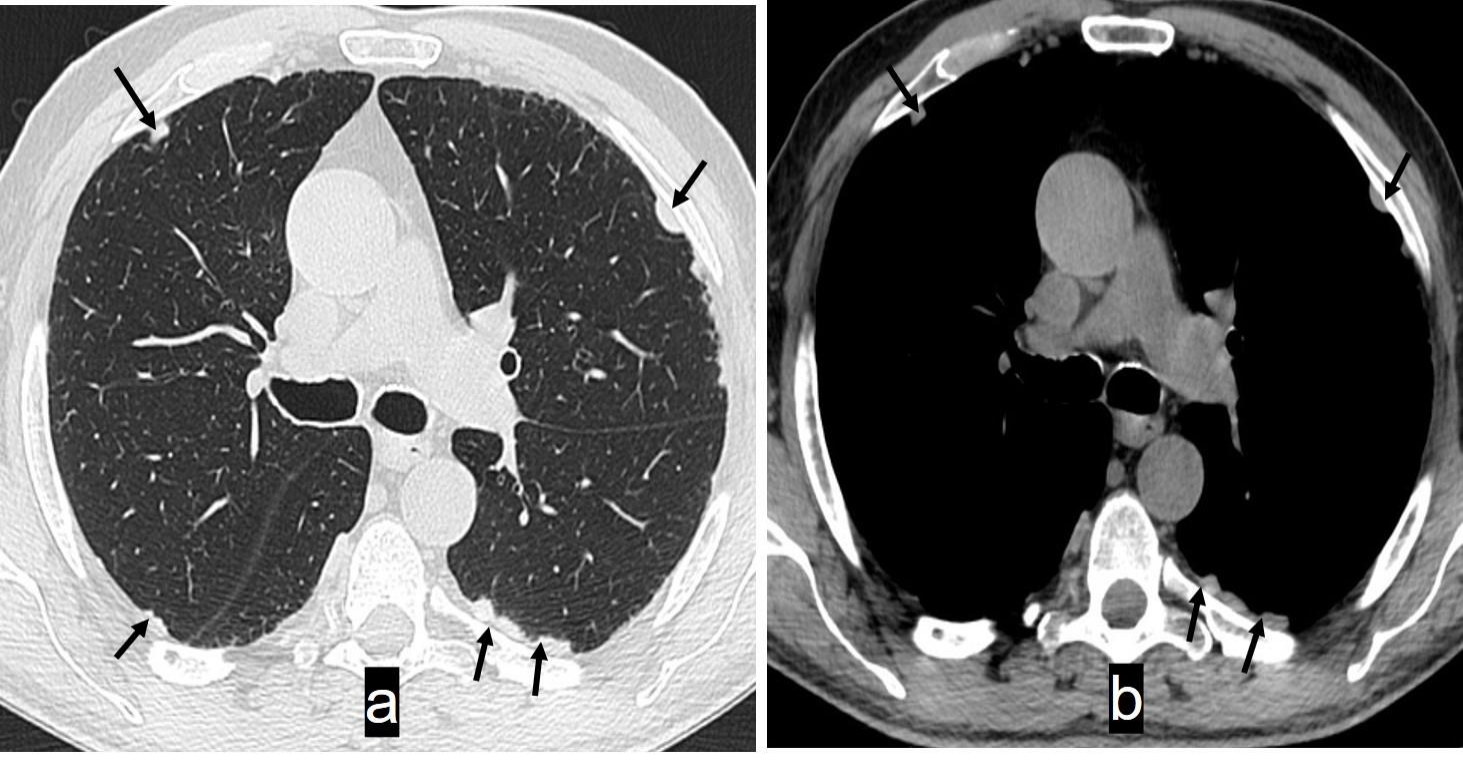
Axial non-contrast CT images of the chest of a 65 years old builder with history of exposure to asbestos show multiple pleural plaques (solid arrows) bilaterally. Plaques are appreciated on the lung (a) and mediastinal (b) windows but their density and size are better delineated on the mediastinal window
